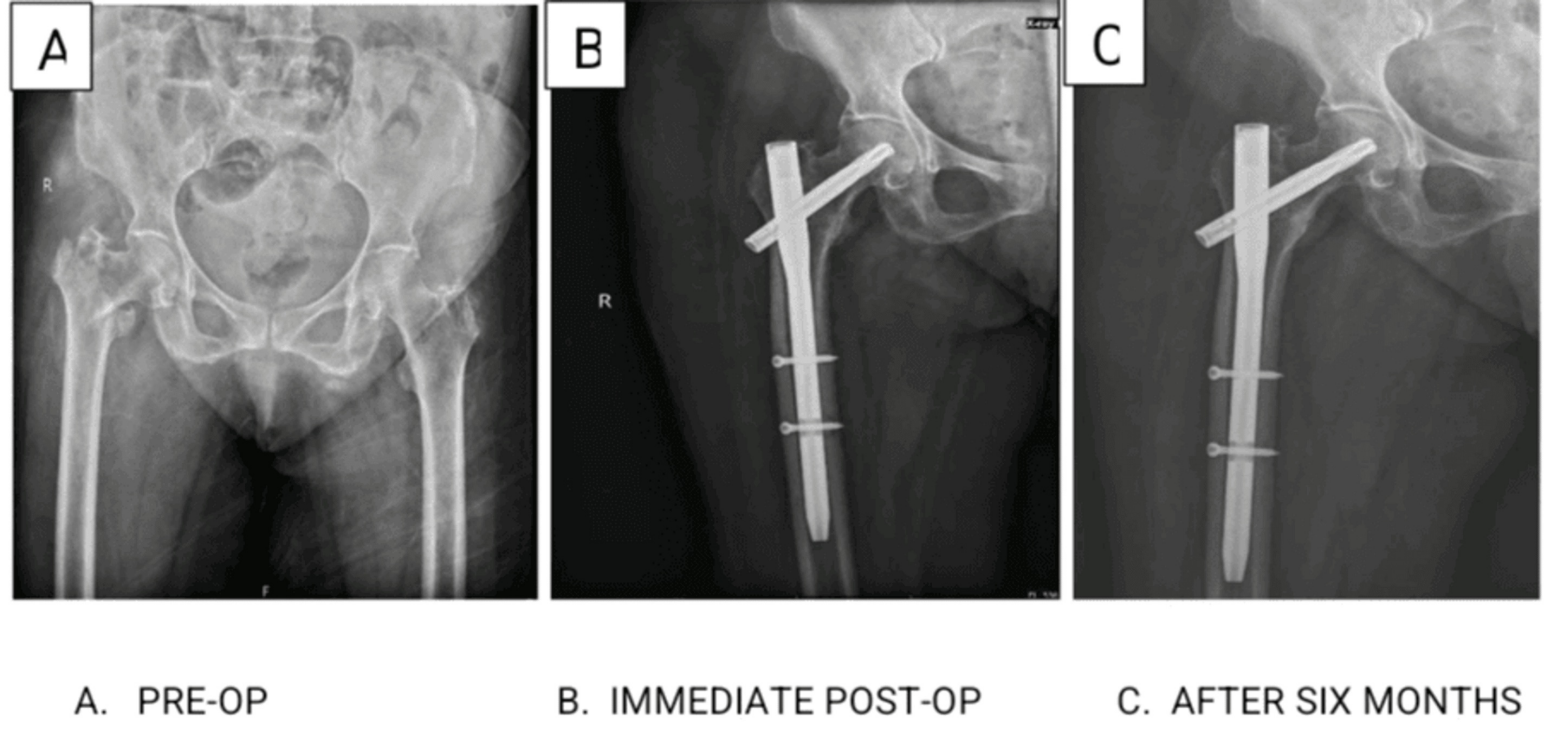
Post-operative X-ray of the patient operated using the proximal femoral nail.
